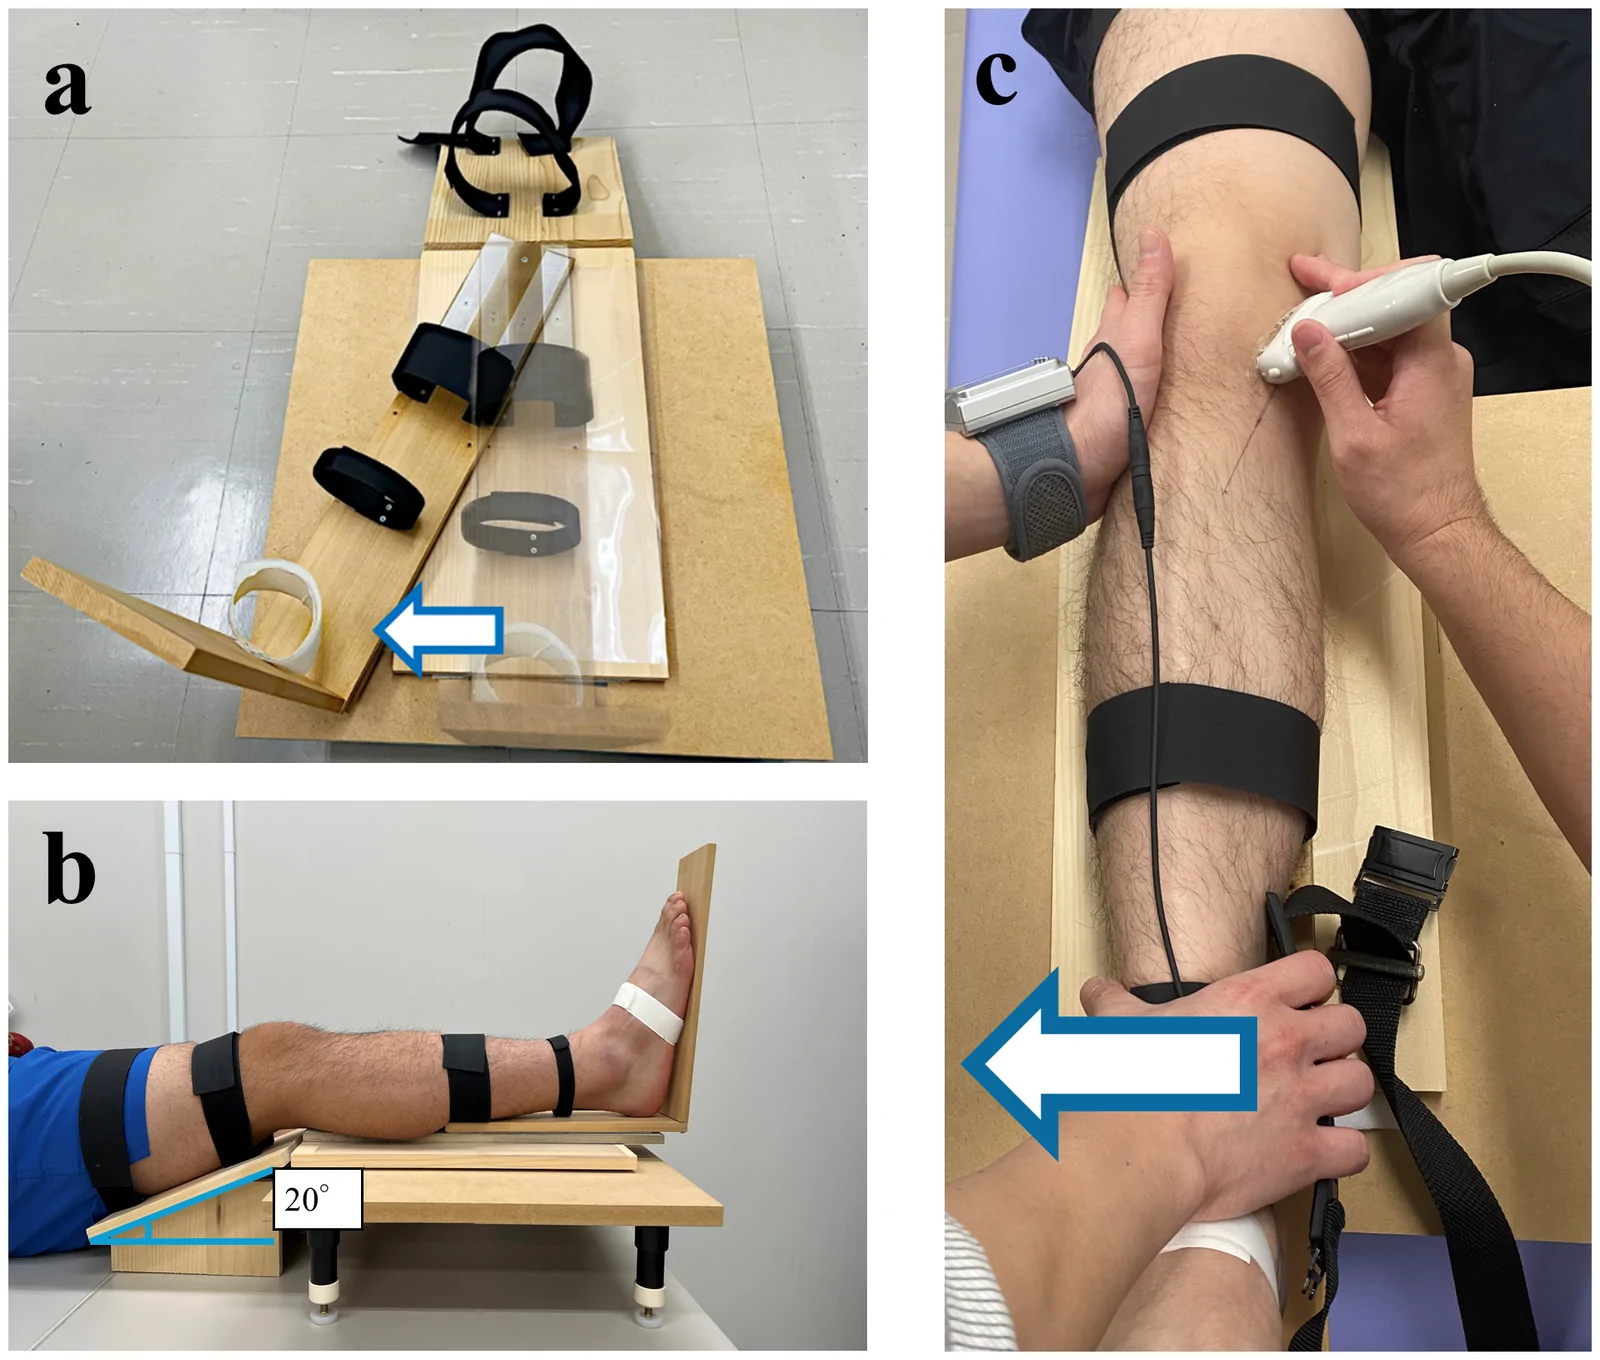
(a) A custom-made fixation device. (b) Knee fixed at 20° of flexion and ankle positioned in neutral dorsiflexion using the device. (c) Measurement of medial knee joint space width using ultrasound imaging.
